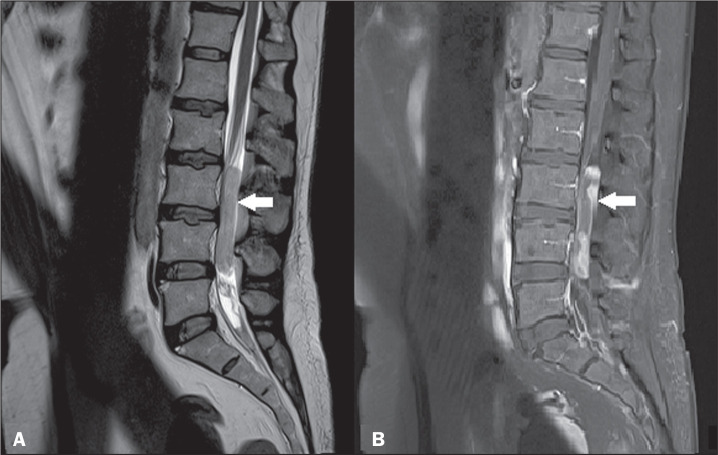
Ependymoma of the filum terminale. Sagittal MRI scans. A: T2-weighted
